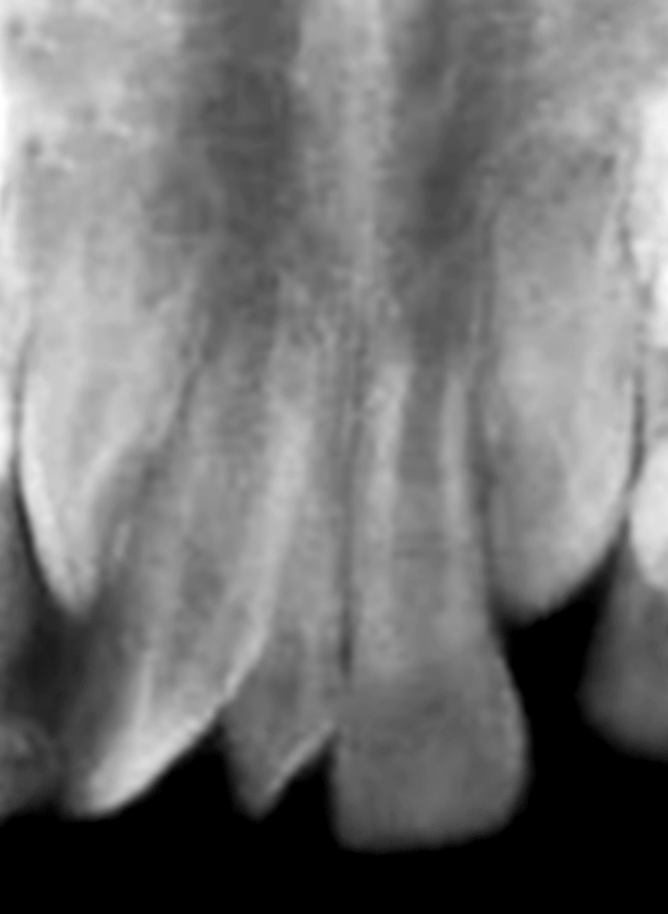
Periapical radiograph showing absence of any other supernumerary teeth
